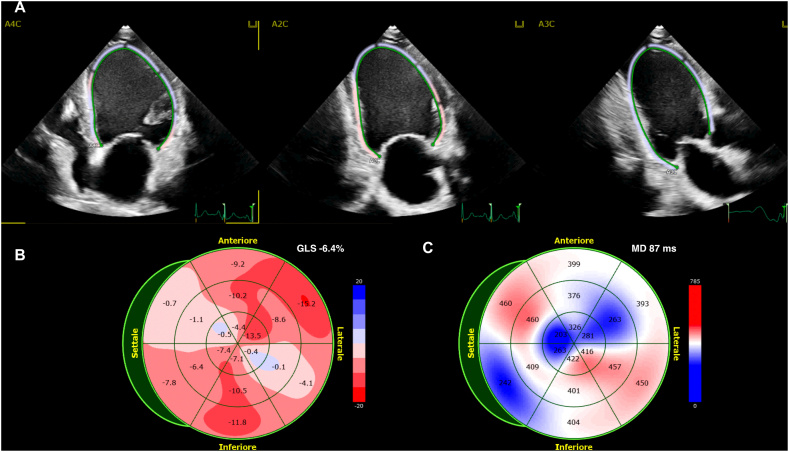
A B C – 1A. Echocardiography based speckle tracking analysis (GLS and MD) in a patient with HF, admitted for sustained VA. 1B. Bull's eye view of segmental longitudinal strain of left ventricle. 1C. Bull's eye view of segmental time to peak systolic strain of left ventricle.
